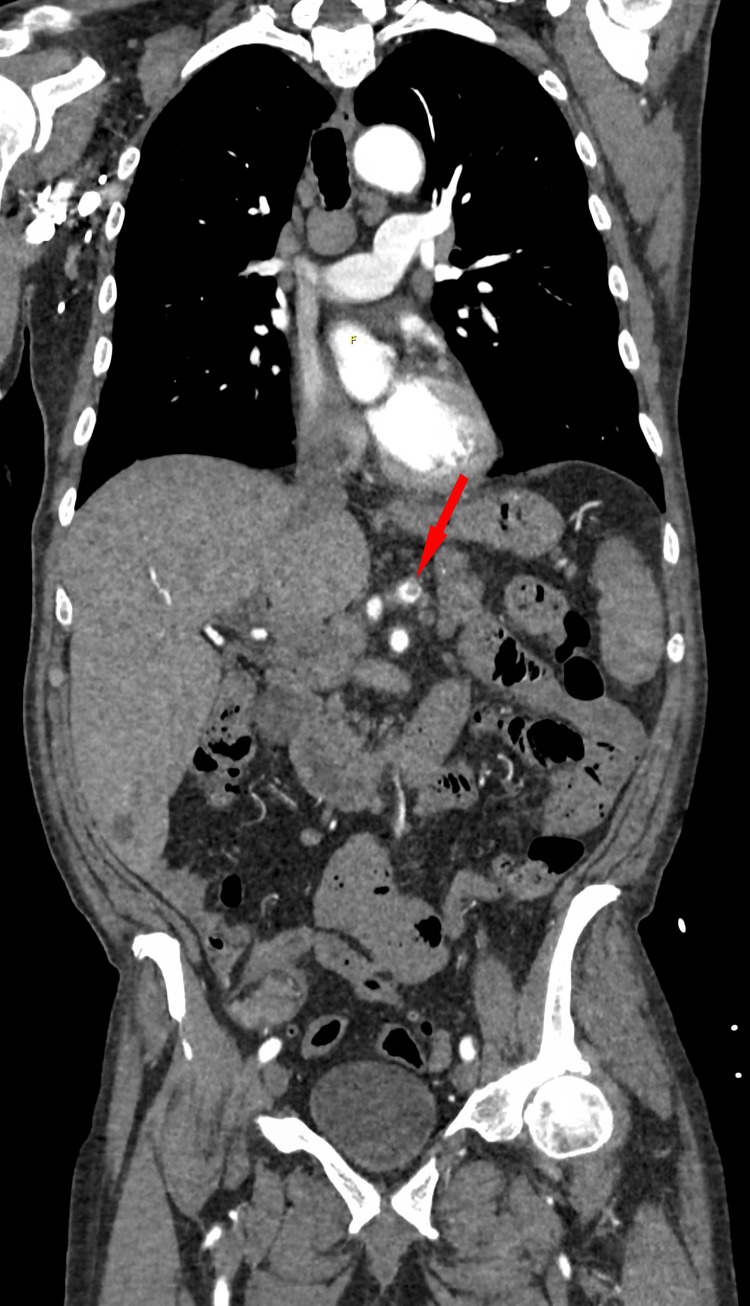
CT angiogram of the chest, abdomen, and pelvis demonstrating celiac artery occlusion due to thrombus (red arrow), and acute splenic infarct. CT, computed tomography
